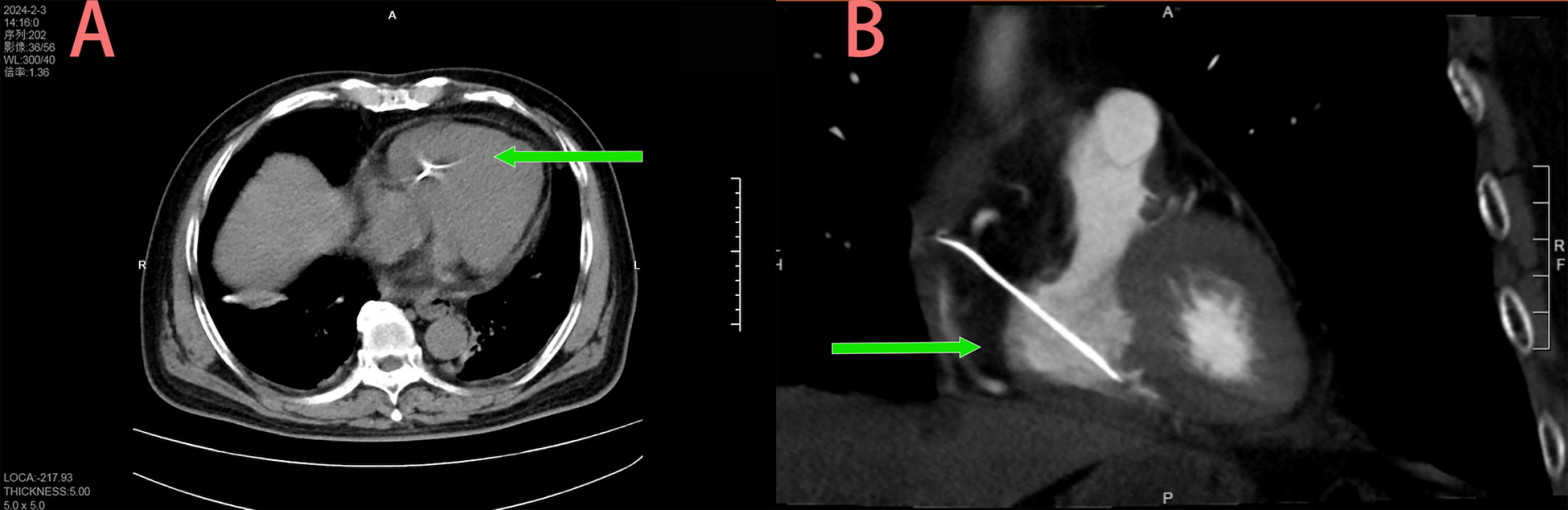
Right intracardiac high density shadow of chest CT (A) and reconstruction (B).
